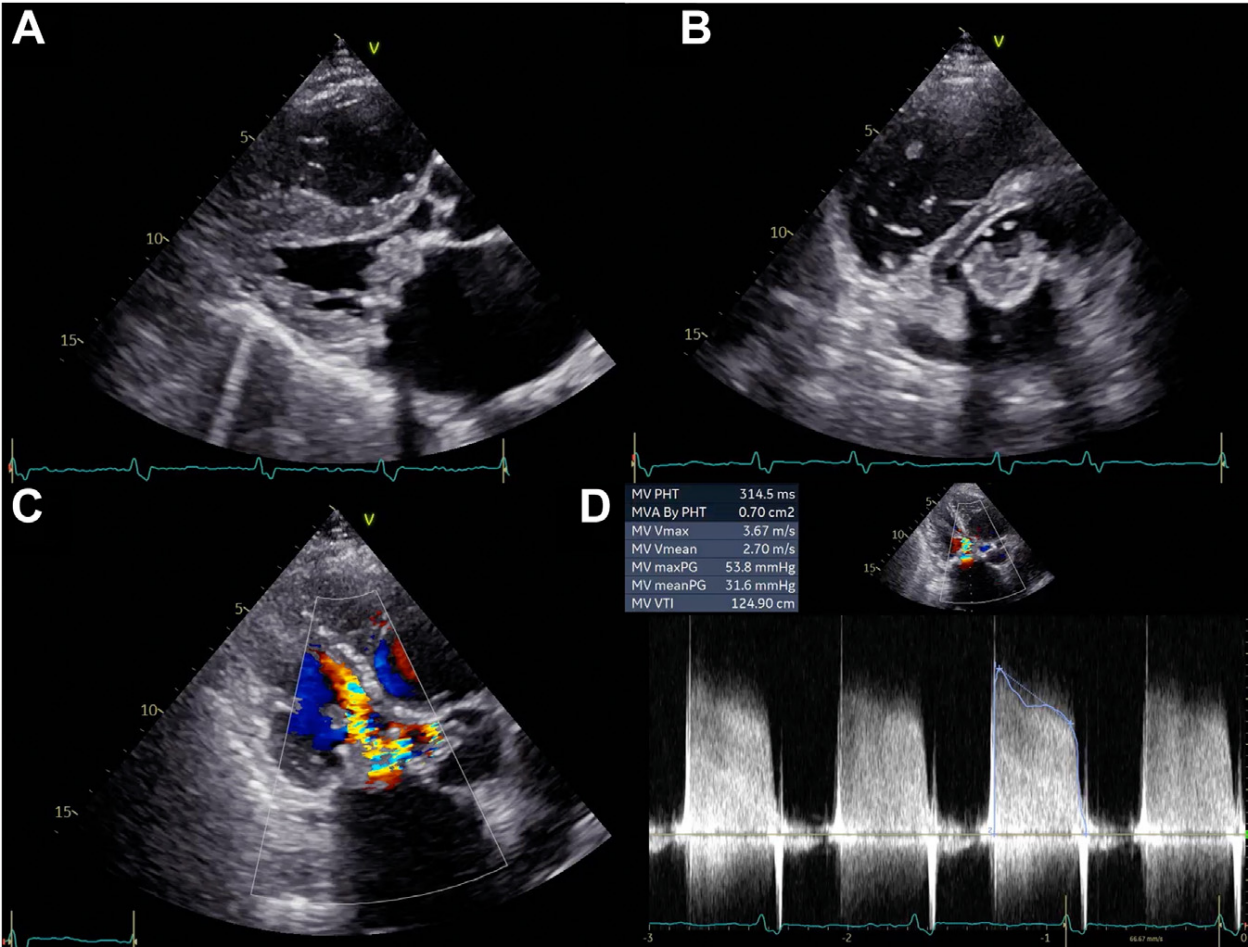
Transthoracic Echocardiographic Images (A and B) Transthoracic echocardiography reveals thickening of the mitral valve (MV) leaflets, (C) no mitral regurgitation at the color Doppler signal, and (D) increased transmitral flow (Video 1). MVA = mitral valve area; PHT = pressure half-time; Vmax = maximum velocity; Vmean = mean velocity; VTI = velocity-time integral.
